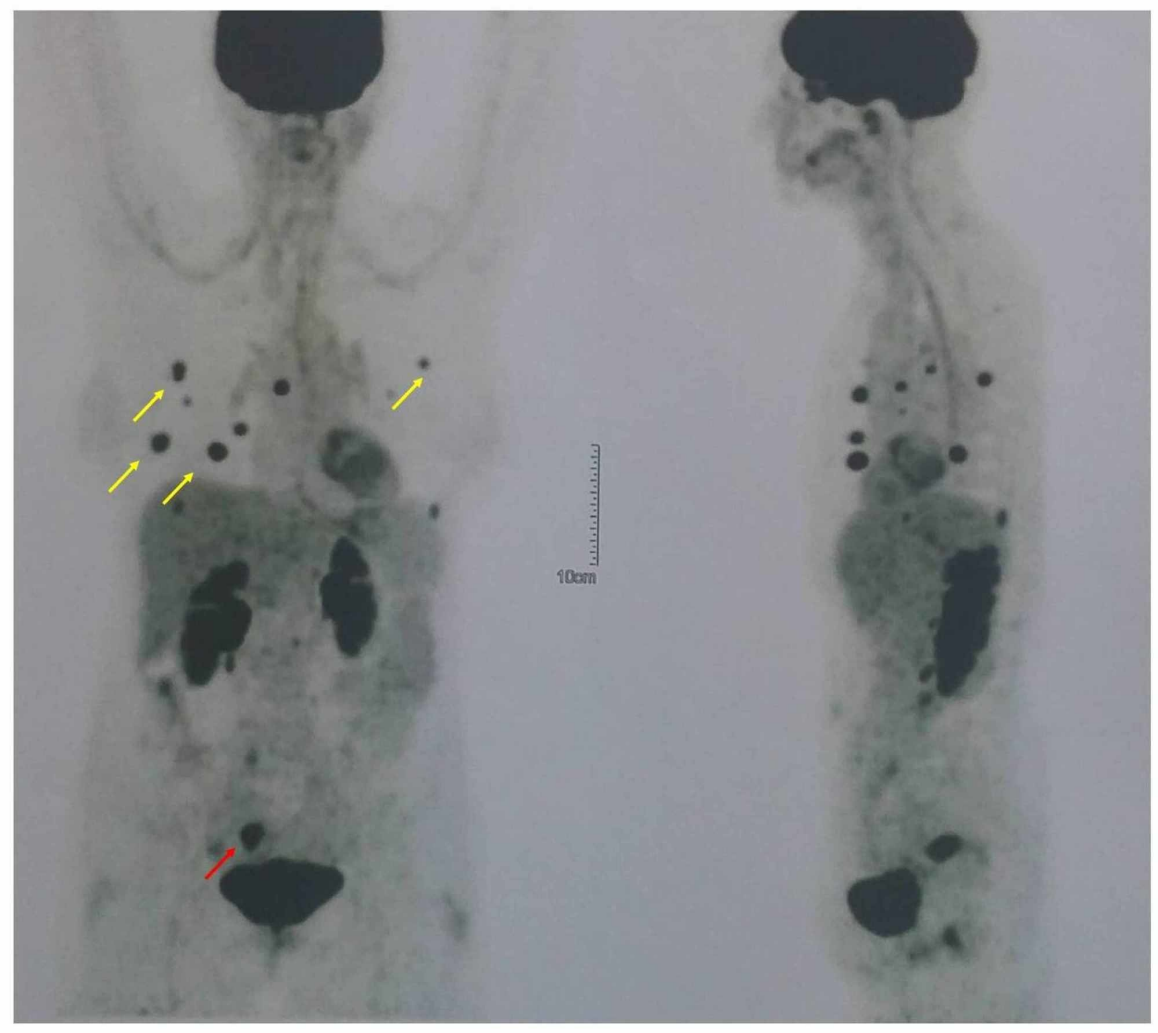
PET-CT scan showing 18F-FDG uptake in the uterus (red arrow) and multiple nodules of both lungs (yellow arrows) PET-CT: positron emission tomography-computed tomography; 18F-FDG: fludeoxyglucose F 18
